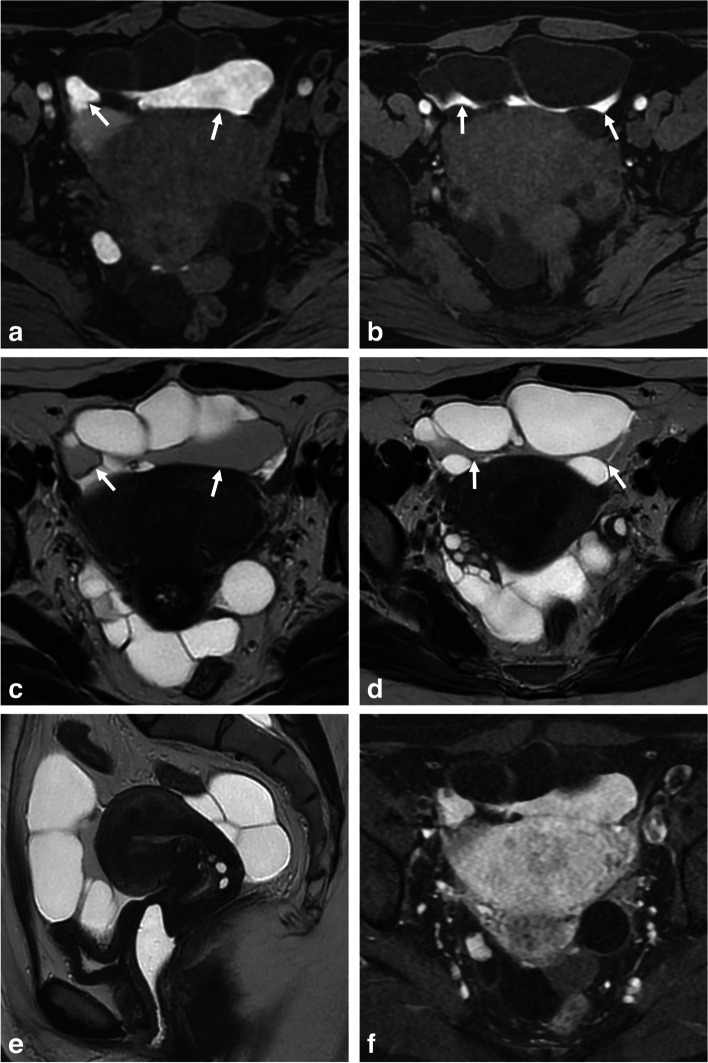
Multicystic peritoneal mesothelioma in a 38-year-old woman with chronic pelvic pain addressed for suspicion of endometriosis. a, b Axial T1-W fat-suppressed MR images show an anterior and posterior pelvic multicystic lesion with some loculi showing hyperintense content (arrows). c, d Axial and (e) sagittal T2-W MR images show a thin-walled multiple grape-like clusters lesion, with intermediate signal intensity in thick-content loculi (arrows), gently molding to the polymyomatous uterus and normal ovaries, as well as the pelvic wall. f Axial fat-suppressed contrast-enhanced subtracted T1-W image shows no thick or irregular wall enhancement of the multicytic lesion, nor tissular portion
